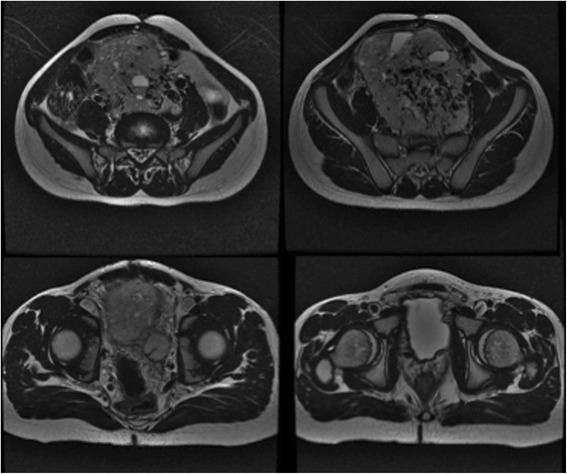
Abdominal MRI revealing a large mass with intense vascularization with close contact aorta and iliac bifurcation
